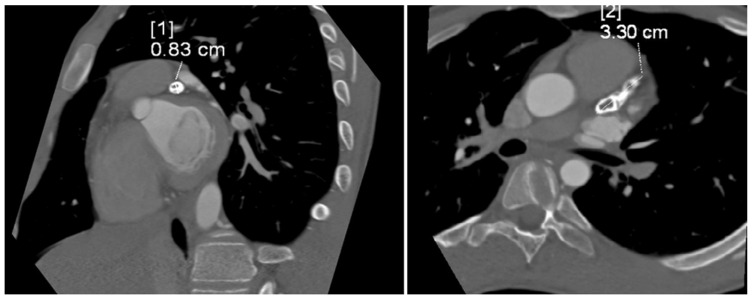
Computed tomography angiogram showing tubular, highly calcified, left coronary aneurysm (8.3 × 15 × 33 mm) without any features of contrasted lumen. Diameter [1]—8.3 mm; diameter [2]—33 mm.
